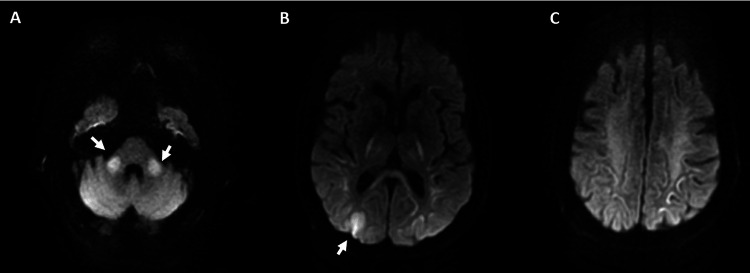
Brain MRI with and without contrast DWI axial images at time of presentation (A) Mild restricted diffusion within the middle cerebellar peduncles bilaterally; (B, C) Increased signal in the right parietal occipital hyperintensity and focal changes within thalami and corona radiata bilaterally. Most likely etiology is posterior circulation ischemia from a hypotensive event.
